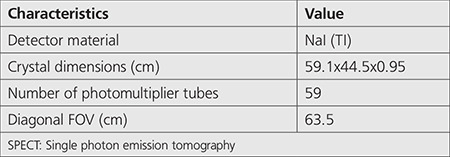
Specifications of the Siemens SYMBIA SPECT scanner
